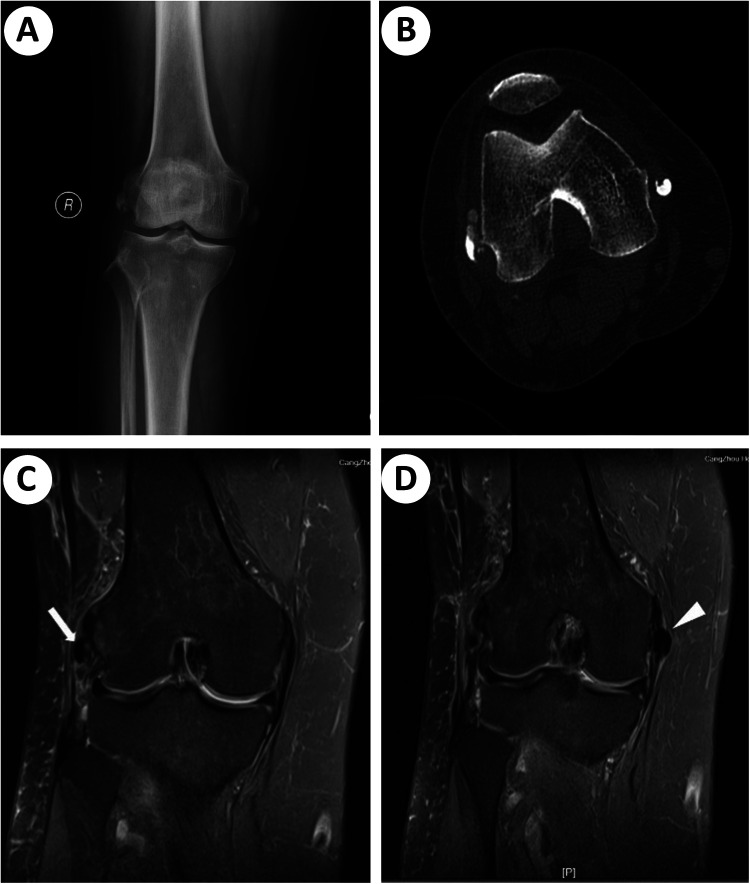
Preoperative radiographic findings. (A) X-ray showing well-defined calcification deposits in close proximity to the bilateral femoral condyles. (B) Axial CT image displaying calcifications. (C) Coronal MRI views of the affected LCL. Zone of hypointense signal (white arrow) suggesting calcific deposit. (D) Coronal MRI views of the affected MCL. Calcification (white arrowhead) adjacent to the proximal insertion of the MCL.
